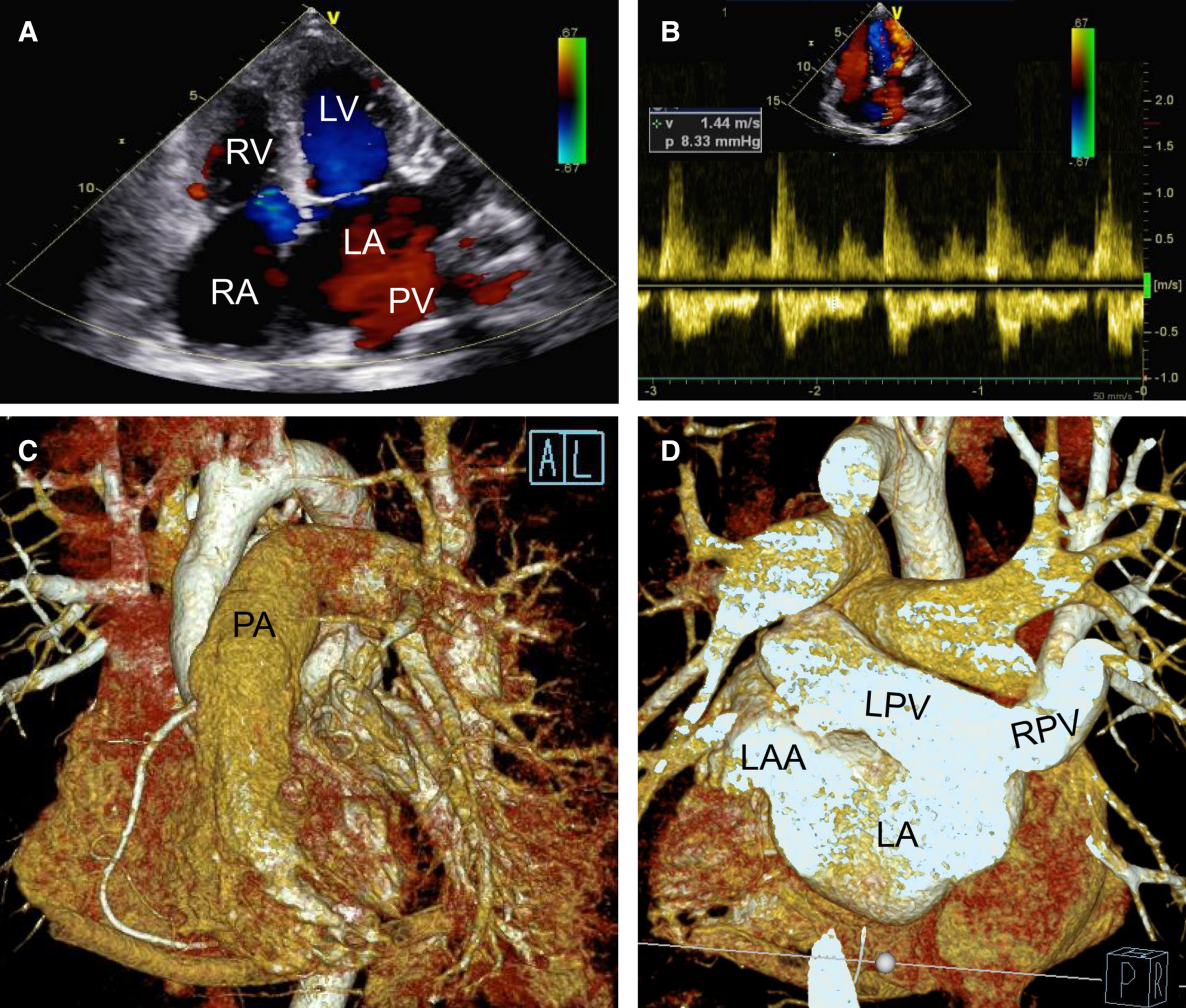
Postoperative transthoracic echocardiography (A,B) and cardiac CTA with three dimensional reconstruction figure (C,D) showing that the deformity correction was satisfactory without residual shunt (A) and pulmonary veinous obstruction (B) but with left and right pulmonary veins connected to the left atrium directly (C,D). LA, left atrium; LAA, left atrium; LPV, left pulmonary vein; LV, left atrial appendage; PA, pumonary artery; PV, pumonary vein; RA, right atrium; RPV, right pulmonary vein; RV, right ventricle.
